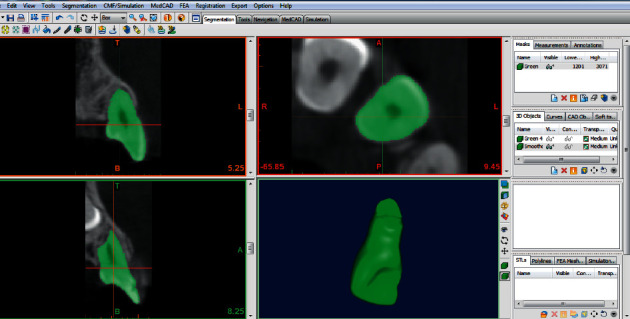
3D view of a severe resorption in lateral root CBCT.
